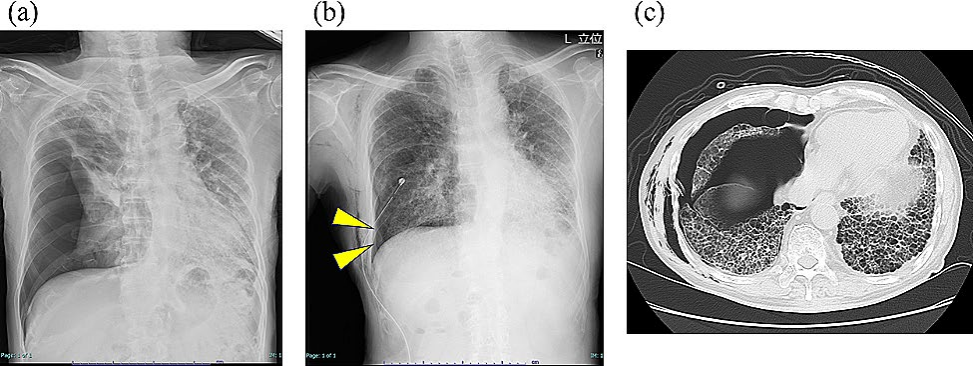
Images taken at admission and post-thoracic drainage. At the emergency visit, the chest X-ray revealed a pneumothorax in the right lung with mediastinal shift (a). After thoracic drainage, a chest X-ray showed a mild pneumothorax cavity (arrowhead) (b), while chest computed tomography revealed collapse of the right middle and lower lobes against a background of ground-glass opacities and honeycombing in both lungs (c)
